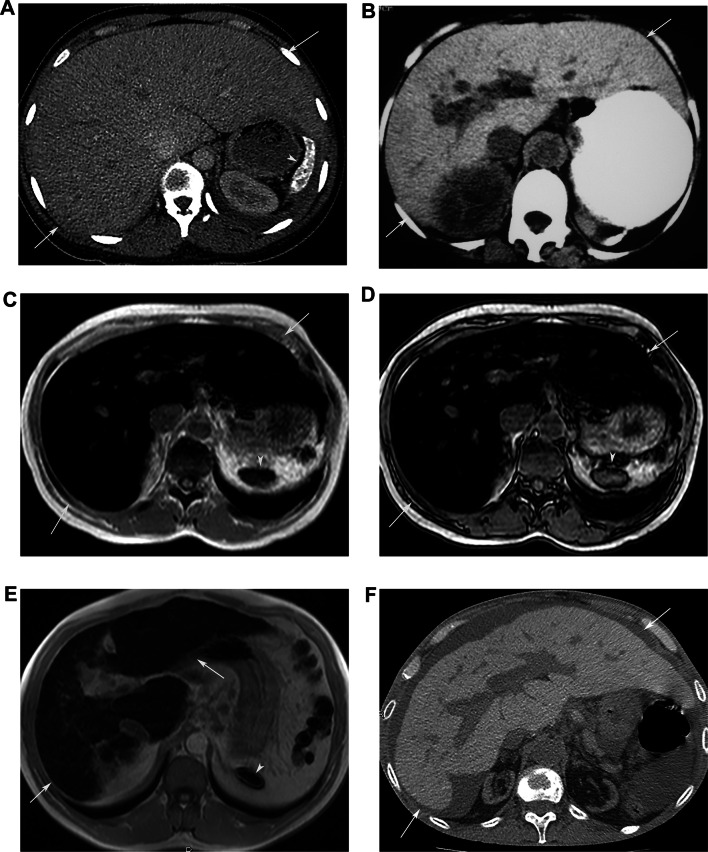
a–f Hepatic manifestations of sickle cell disease. a Post-contrast CT scan of a 26-year-old patient with sickle cell disease presenting with a diffuse pain crisis predominantly affecting the limbs, two recent episodes of fever, and choluria associated with worsening jaundice. The scan demonstrates hepatomegaly due to hepatic congestion (arrows). Evidence of autosplenectomy is also noted (arrowhead). b Non-contrast CT scan of a 41-year-old patient with long-standing sickle cell disease demonstrating diffusely increased hepatic attenuation (arrows), consistent with secondary hemochromatosis. c, d Axial T1-weighted in-phase (c) and out-of-phase (d) MRI images of a 52-year-old patient with long-standing sickle cell disease. The images demonstrate diffuse hypointense signal in the liver (arrow) and spleen (arrowhead), with a marked signal drop on in-phase imaging, findings suggestive of iron overload. e T1-weighted MRI of a 51-year-old male with sickle cell disease, presenting with jaundice, disorientation, and somnolence. The image demonstrates features of chronic liver disease, including relative hypertrophy of segments II, III, and the caudate lobe; hypotrophy of segment IV; irregular hepatic contours; and widened fissures (arrows). Diffuse low signal intensity of the liver reflects iron deposition. Evidence of autosplenectomy is also seen (arrowhead). f Non-contrast CT scan of a 62-year-old male with sickle cell disease, presenting with dyspnea on minimal exertion and orthopnea. Past medical history includes priapism, sickle cell nephropathy, and osteonecrosis of the femoral head. The image demonstrates features of chronic liver disease, including irregular hepatic contours and hypertrophy of the left and caudate lobes (arrows). Ascites is also present
